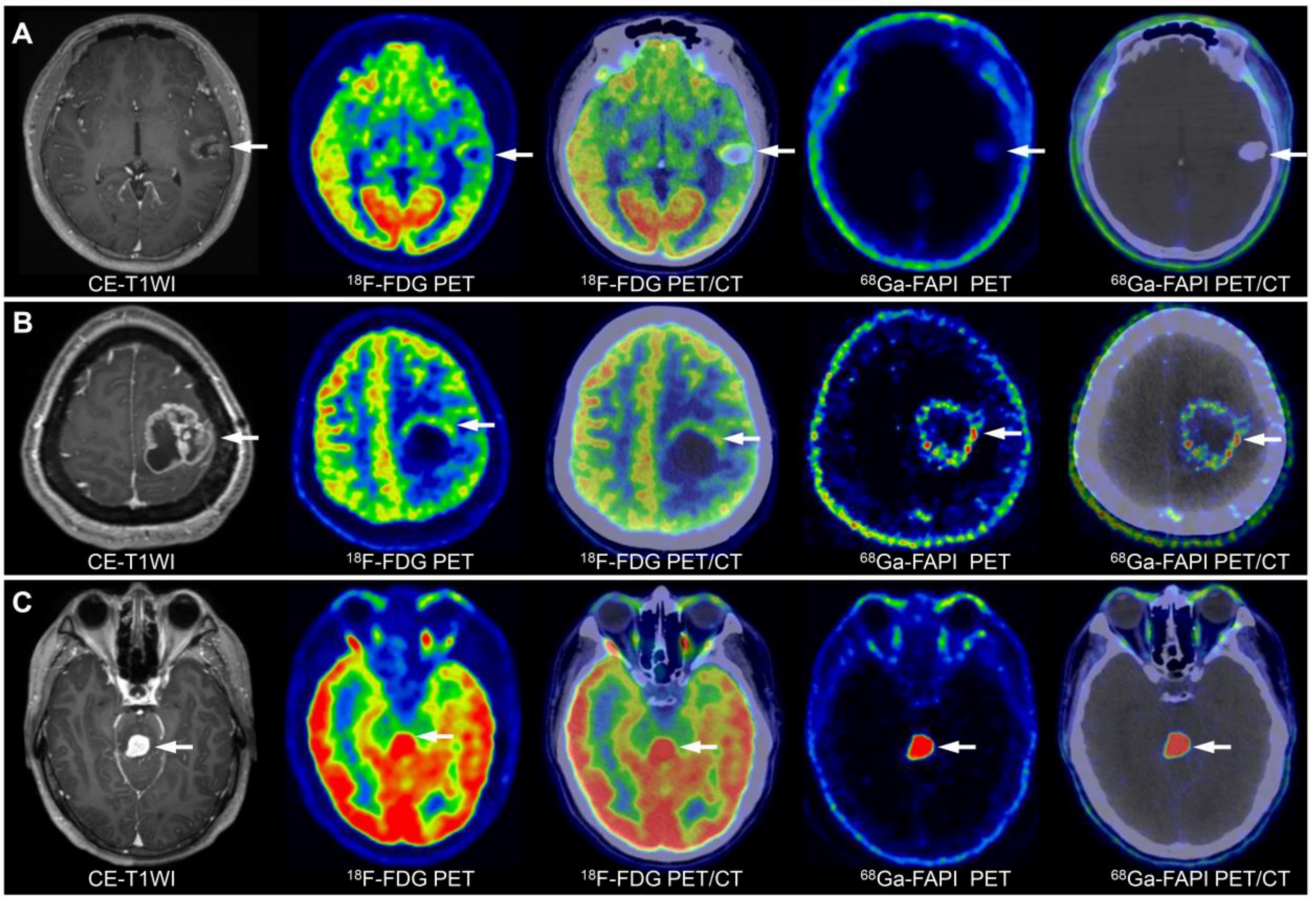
Image characterisation of different WHO grades of gliomas on ^68^Ga-FAPI-46 PET, ^18^F-FDG PET, and CE-MRI (tumour lesions are indicated by the arrows). A 15-year-old male with a low-grade oligodendroglioma in the left temporal lobe showed mild ^18^F-FDG and ^68^Ga-FAPI-46 uptake (A). A 45-year-old female with a WHO grade III glioma in the left parietal lobe exhibited ring-shaped elevated ^68^Ga-FAPI-46 uptake, with locally uneven increased ^18^F-FDG at the margin (B). A 36-year-old male with a WHO grade IV glioma in the brainstem demonstrated significantly increased tracer uptake on both ^68^Ga-FAPI-46 and ^18^F-FDG PET/CT (C).
